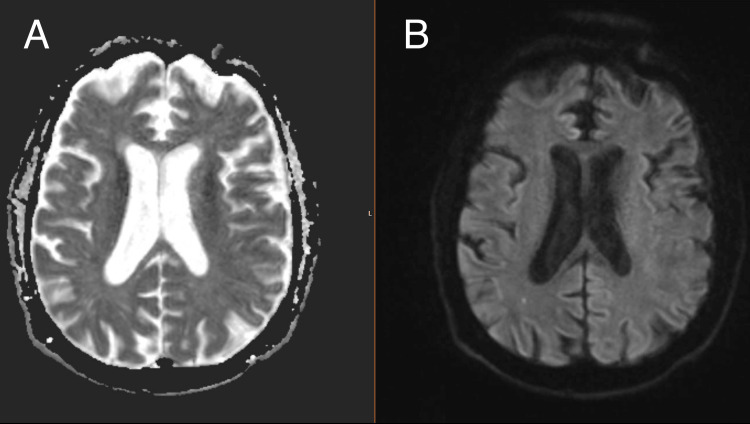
Magnetic resonance imaging of the brain demonstrating subacute embolic infarcts (A) Apparent diffusion coefficient (ADC) sequence demonstrating no corresponding abnormal signal in the right parietal lobe. (B) Diffusion-weighted imaging (DWI) sequence demonstrating a small hyperintense focus in the right parietal lobe. The presence of DWI hyperintensity without a corresponding abnormality on ADC is consistent with a subacute infarct in the right parietal lobe.
